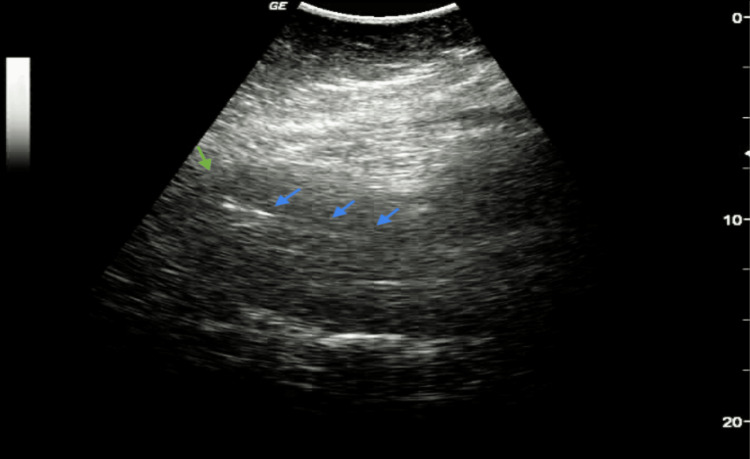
Abdominal control ultrasound. blue arrows: clean endometrial lining; green arrow: uterine fundus
